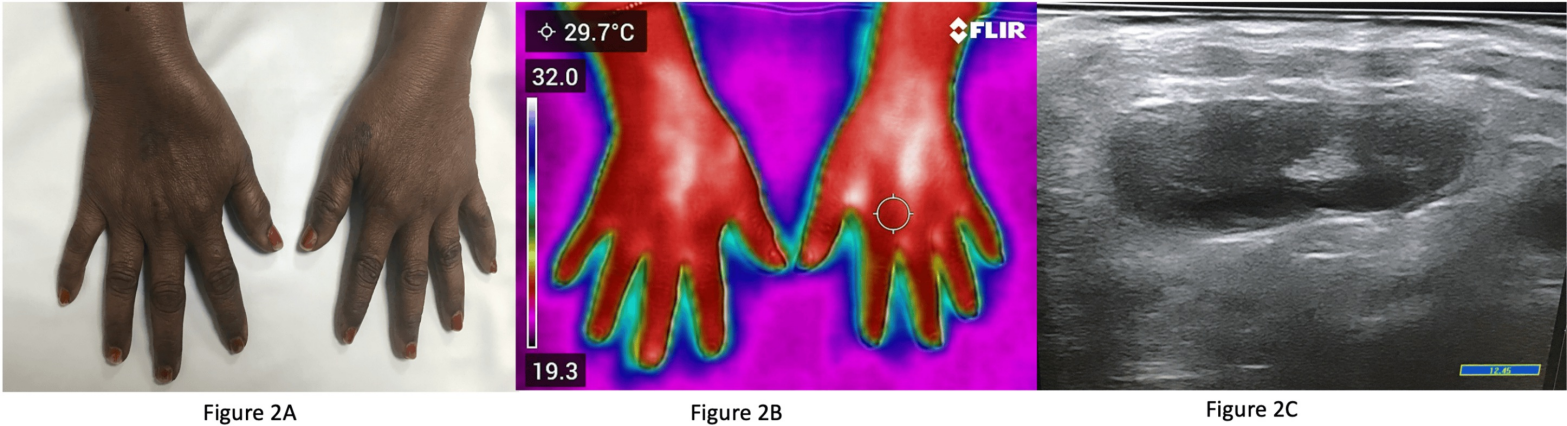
Image of a patient's hands 2A: Clinical image of a patient with swelling over the bilateral wrists extending across the joint line involving the extensor tendons; 2B: Infrared thermography of the same patient demonstrating increased temperature, which is distributed across the extensor tendon; 2C: Ultrasonography of the same confirming tenosynovitis.
